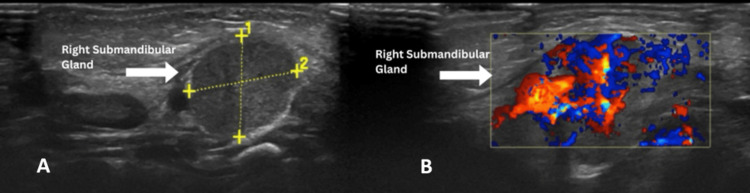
Ultrasound of the right submandibular gland. A: a mildly bulky right submandibular gland measuring 1.2 × 1.1 cm, hypoechoic with evidence of mild capsular thickening and adjacent fat stranding (arrow), no evidence of duct dilatation and calcification; B: increased vascularity and pericapsular inflammation (arrow).
